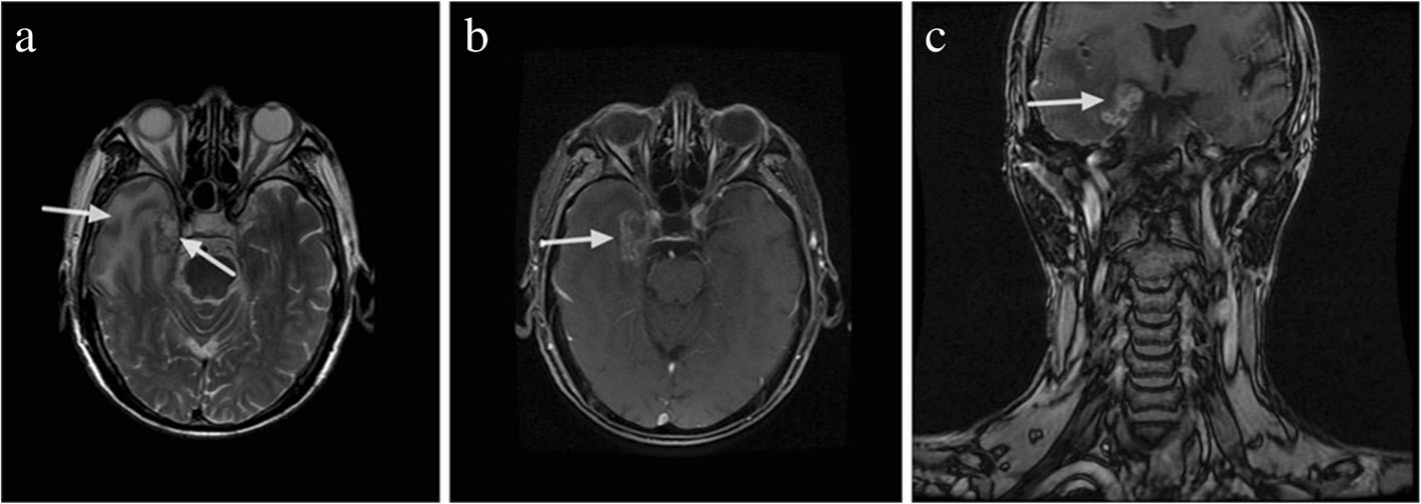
An example of temporal lobe injury showing (a) white matter lesions with a high signal intensity on T2-weighted images, (b) contrast-enhanced lesions on post-contrast T1-weighted images, and (c) lesions on the sagittal MR images
